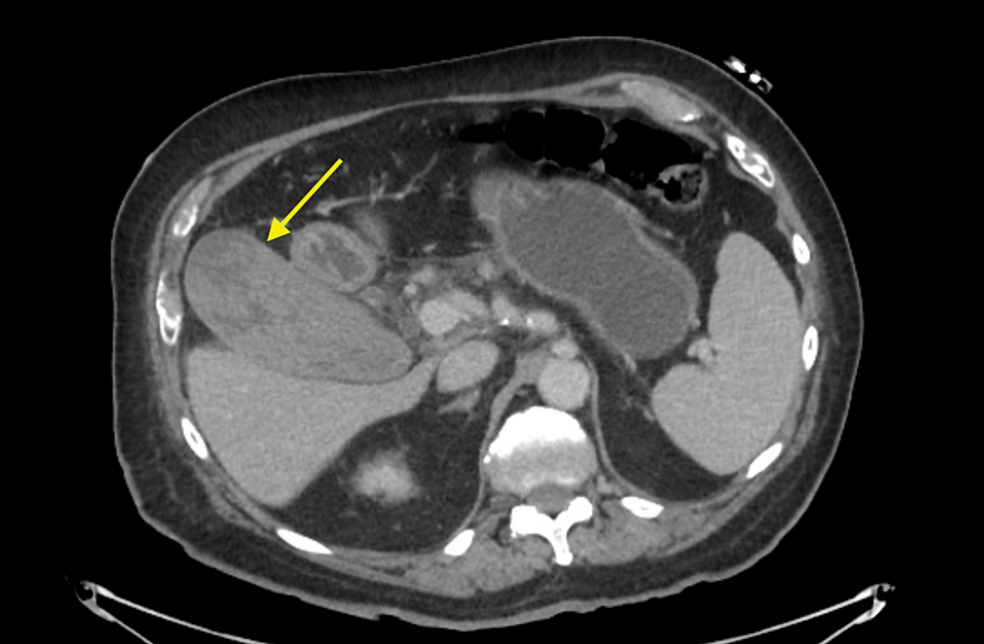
Preoperative CT pancreas protocol demonstrating a distended gallbladder with mixed hyperdensities.
